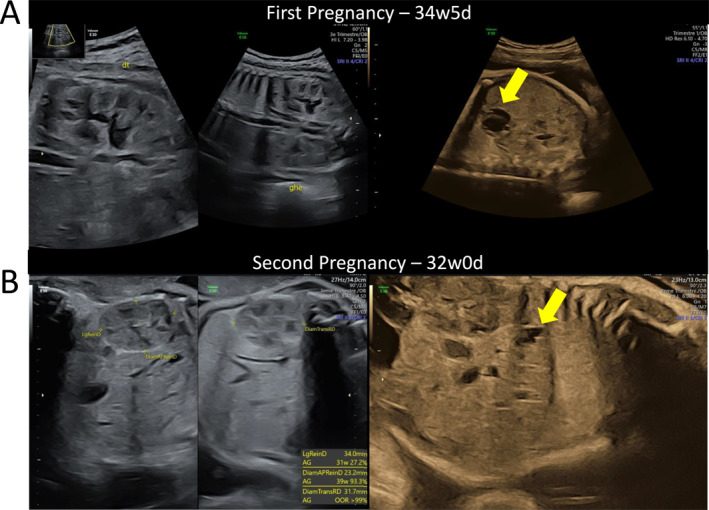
Prenatal ultrasound images from the two consecutive pregnancies showing bilateral kidneys on the left side and the liver on the right side. (A) First pregnancy at 34 + 5 GA: heterogeneous, hepatic non‐vascularized cystic‐solid mass (32 × 23 mm, arrow) (B) second pregnancy at 32 GA: multiple hepatic anechoic lesions (largest 12 × 4 mm, arrow). Kidneys increase in volume.
